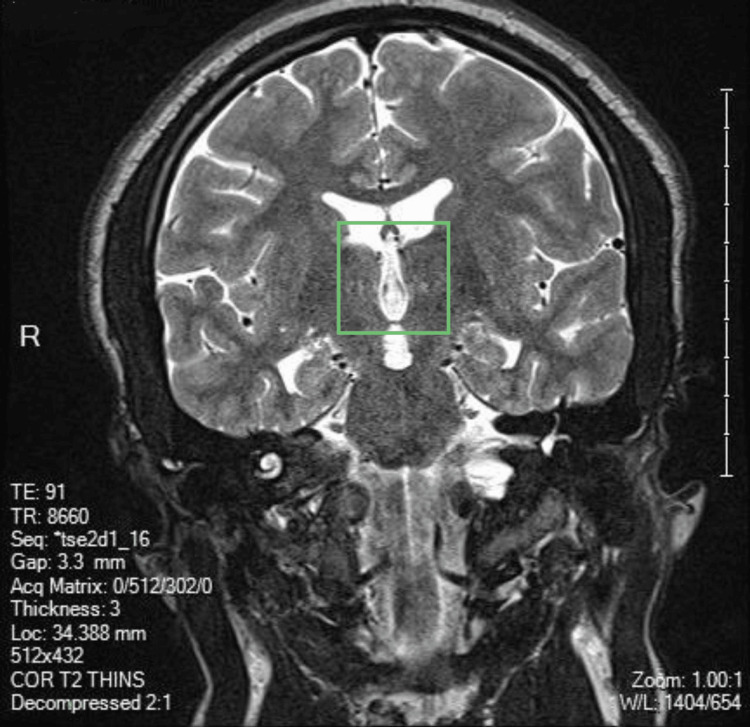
Coronal T2-weighted MRI. Coronal T2-weighted MRI. The third ventricle is indicated within the green rectangle. The image demonstrates symmetric hemispheric structures with no additional associated structural abnormalities at this level, complementing the findings of the posterior CSF-filled space.
